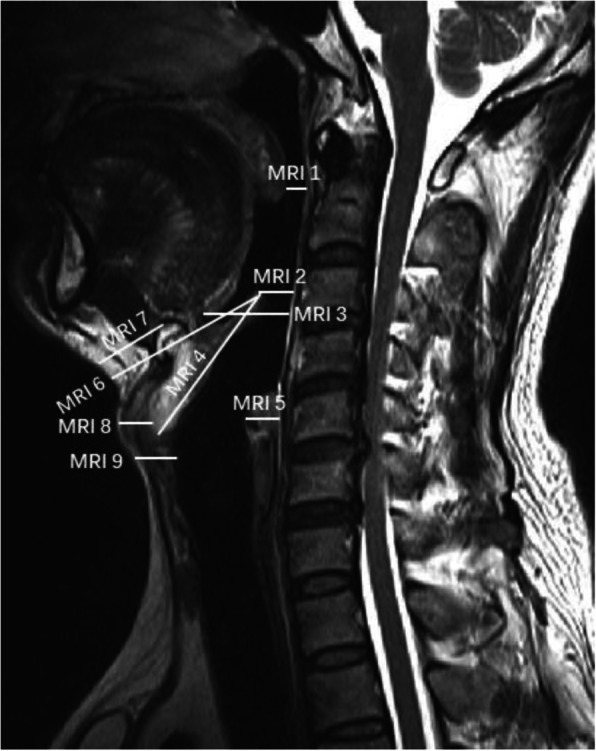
Distance indicators on the lateral sagittal neck MRI in the neutral position. MRI 1: distance between uvula and the posterior pharyngeal wall; MRI 2: distance between the tip of epiglottis and the posterior pharyngeal wall; MRI 3: distance between the base of tongue and the posterior pharyngeal wall; MRI 4: the length of epiglottis; MRI 5: distance between vocal cord and the posterior pharyngeal wall; MRI 6: distance from skin to the tip of epiglottis; MRI 7: distance from skin to hyoid bone; MRI 8: distance from skin to thyroid cartilage at the level of vocal cord; MRI 9: distance from skin to vocal cord
